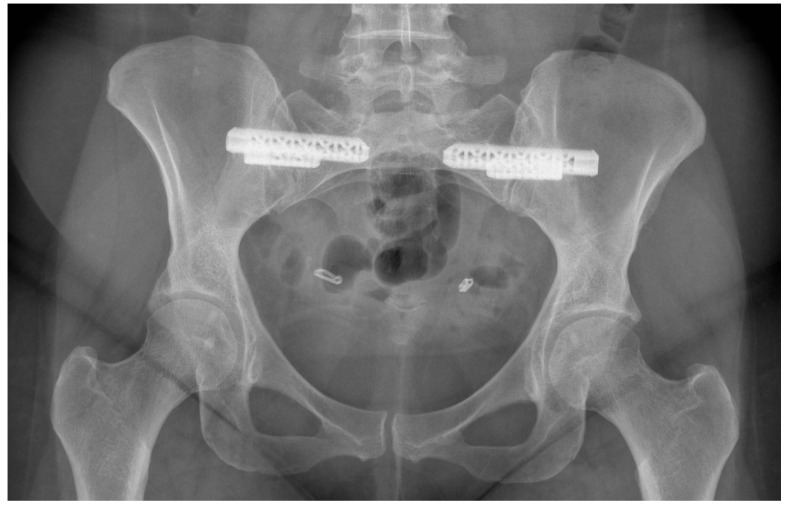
Anteroposterior pelvic radiograph in a 38-year-old female with hypermobility syndrome suffering from chronic sacroiliac joint pain who underwent stabilisation of bilateral sacroiliac joints with the iFuse implants.
